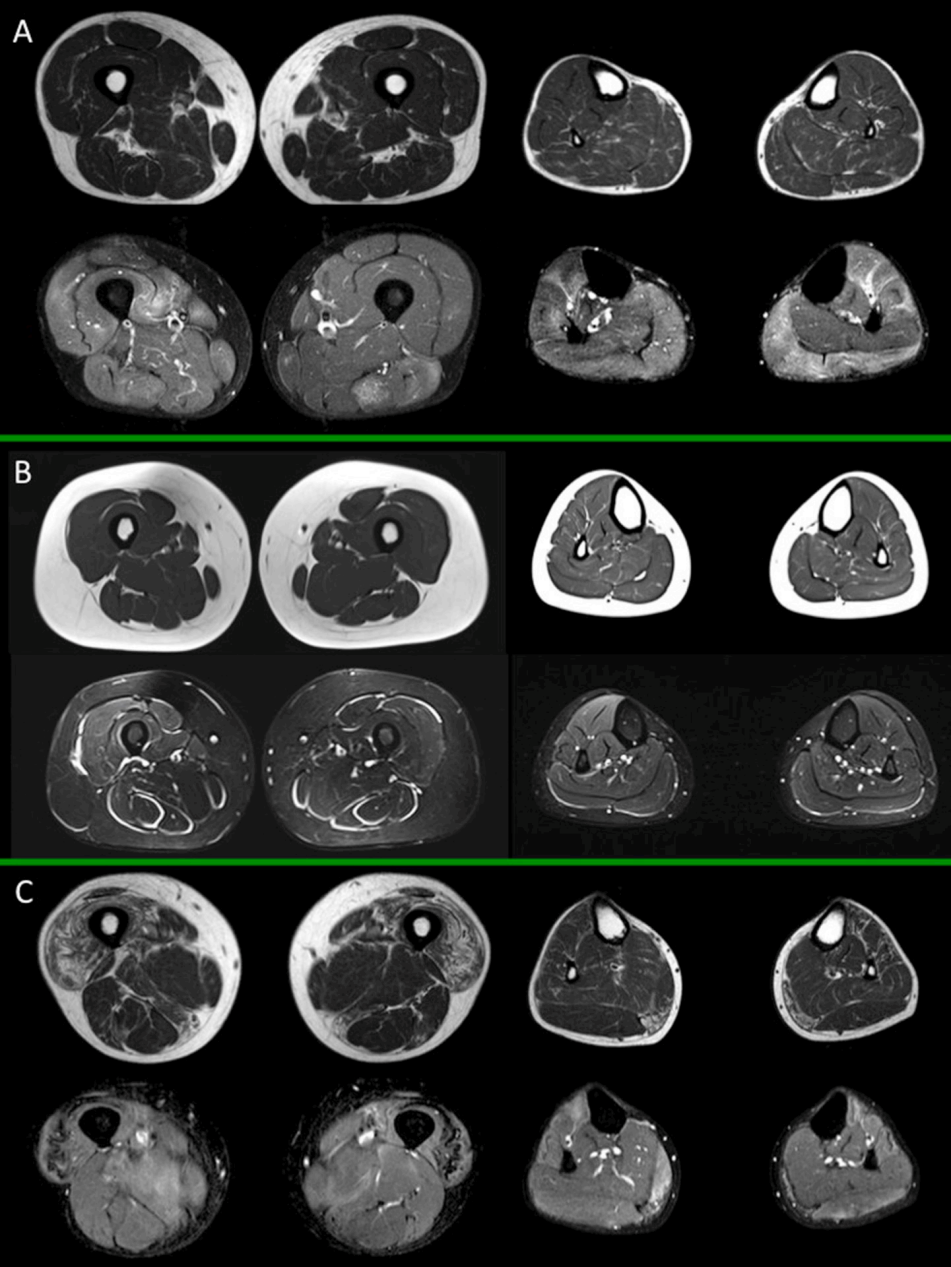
Muscle MRI in inflammatory myopathies. For each box, thighs are displayed on the left, legs on the right side; spin-echo T1-weighted images are on top, short-tau inversion recovery (STIR) images on the bottom. (A) Adult subject with polymyositis (PM), showing multifocal muscular edema involving both thigh and leg muscles and no selective pattern of fat replacement; (B) Adult subject with dermatomyositis (DM) showing characteristic multifocal perifascicular edema involving both thigh and leg muscles; (C) Adult subject with inclusion body myositis (IBM), with predominant fatty substitution of anterior thigh musculature and gemini muscles, associated to slight multifocal muscle edema. All presented MRI images have been collected during routine patient care.
